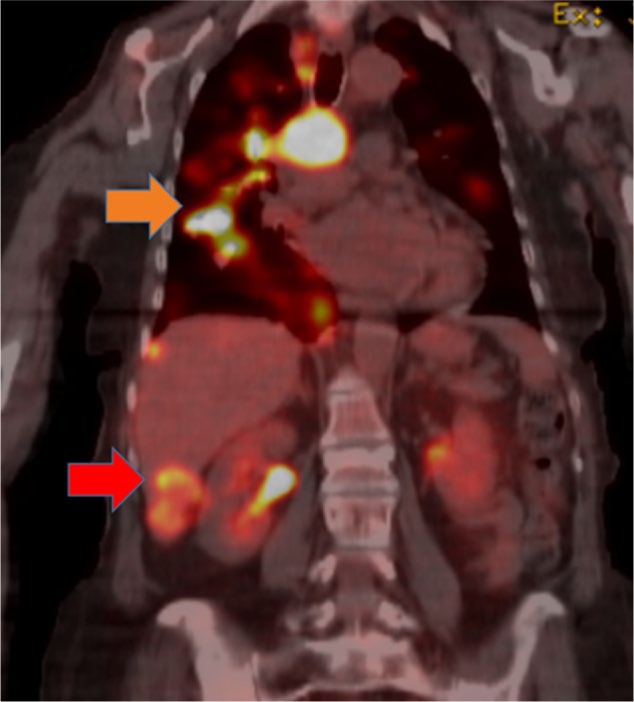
PET-CT scan shows both pulmonary and hepatic disease progression (orange and red arrows, respectively).
